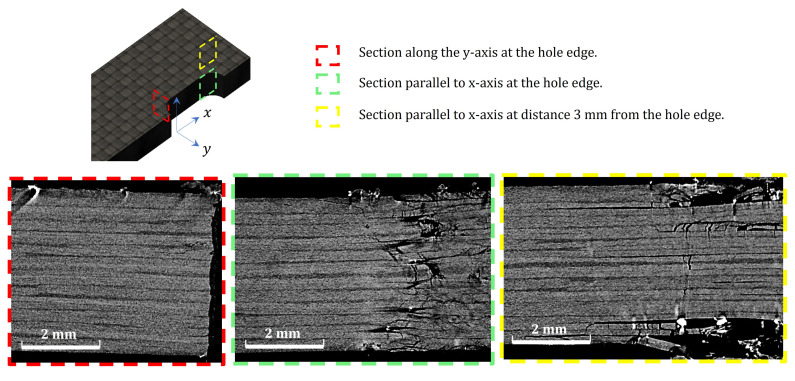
Computed Tomography scan of the baseline laminate.
